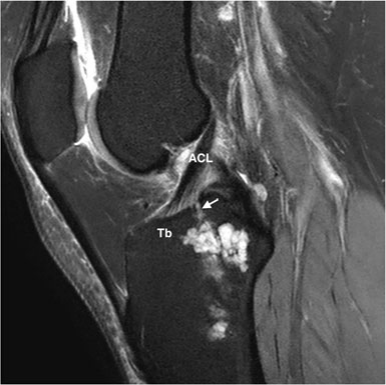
Intraosseous ganglion cyst of the tibia incidentally depicted in a 40-year-old man who underwent an MRI scan due to intermittent, subacute non-specific knee pain. Sagittal FS PD-WI shows a metaepiphyseal, large, multiloculated cystic lesion of the tibia, which communicates with the articular surface through a thin stalk (arrow) extending into the interspinous region, close to the anterior cruciate ligament tibial insertion. Tb, tibia; ACL, anterior cruciate ligament
